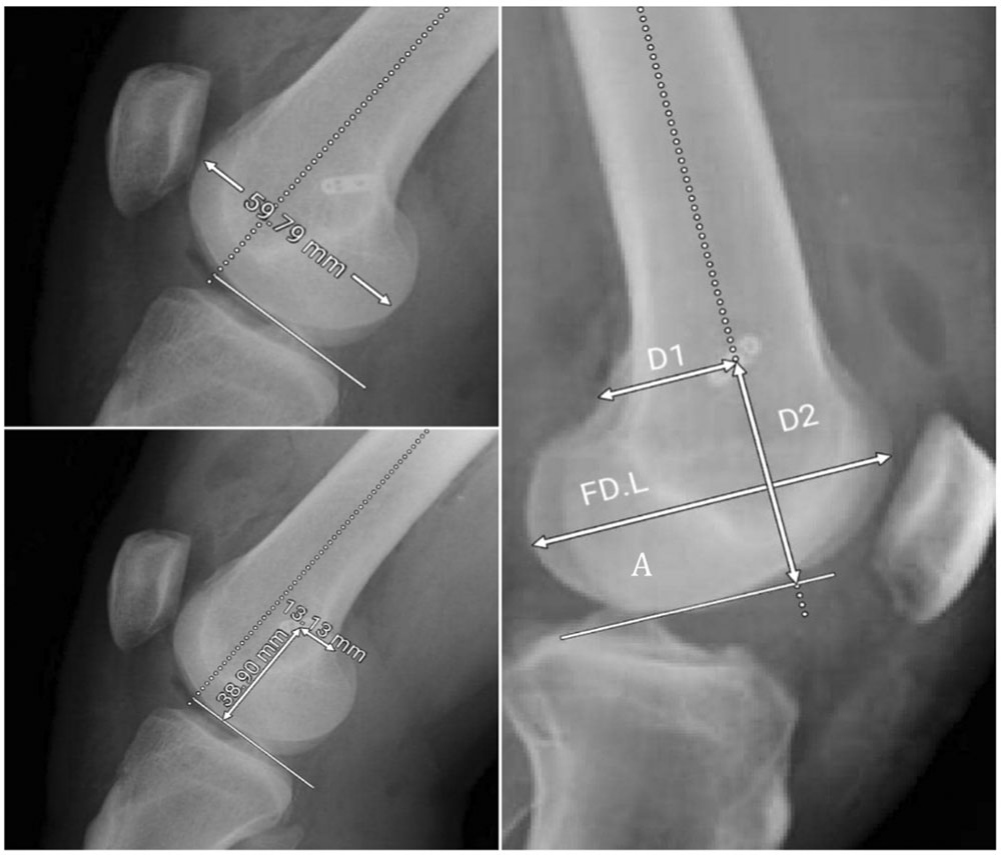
Features of lateral radiographs.
